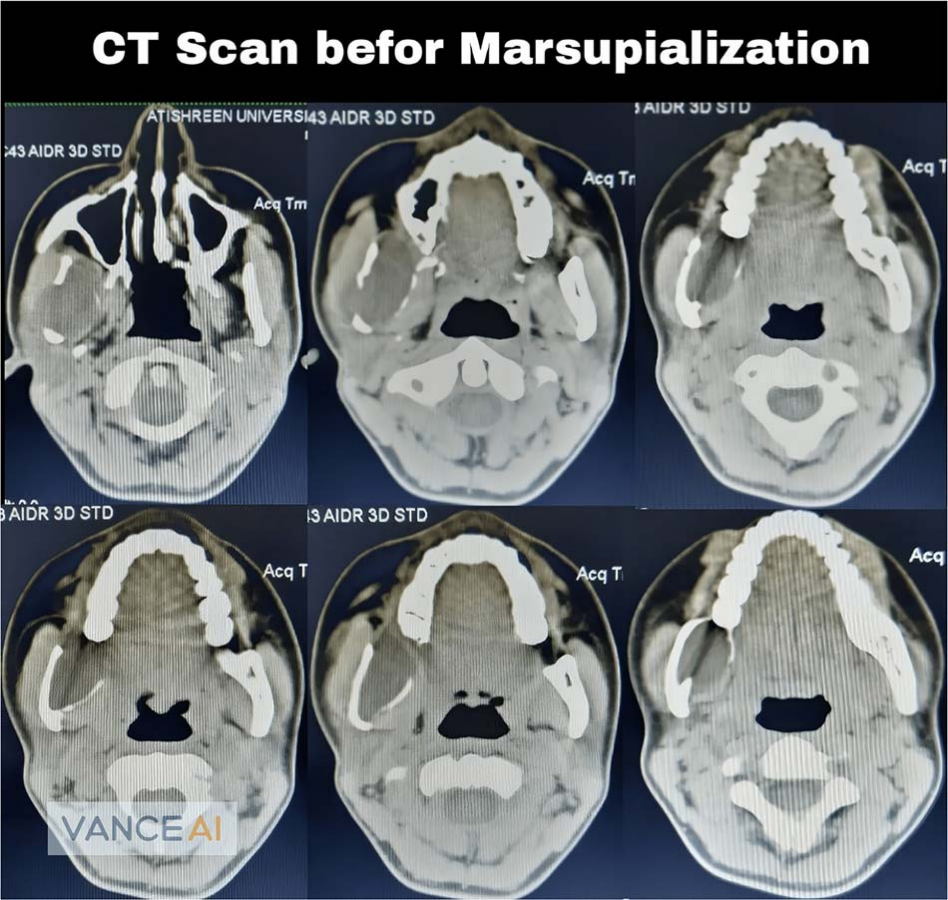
Computed tomography scan showed a cavity in the right mandibular ramus with expansion and absence of most buccal and lingual bone plates.
